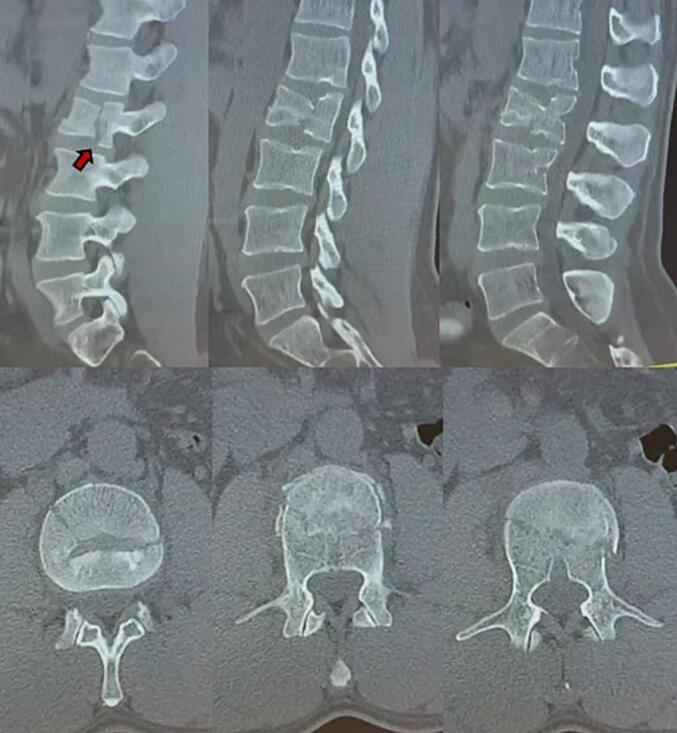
Computed Tomography (CT) scan of vertebral column, immediately after the trauma (sagittal and transverse views). Red arrow highlights the L2 vertebral compression fracture, with voluminous anterior bone fragment. (For interpretation of the references to colour in this figure legend, the reader is referred to the web version of this article.)
